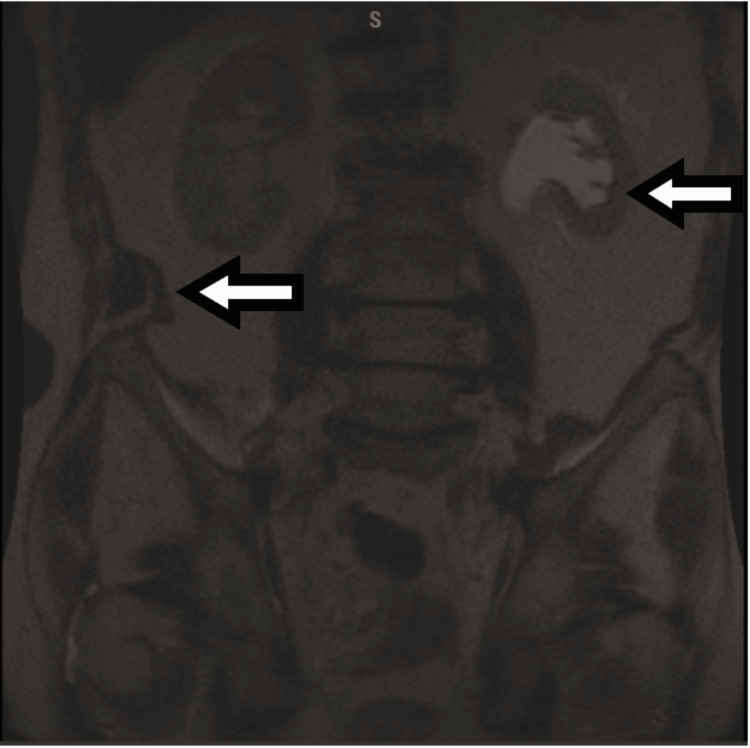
MRI of the spine Coronal view showing moderate to severe left hydroureteronephrosis, a nonspecific 11 mm cystic focus in the right iliacus muscle. MRI: magnetic resonance imaging
